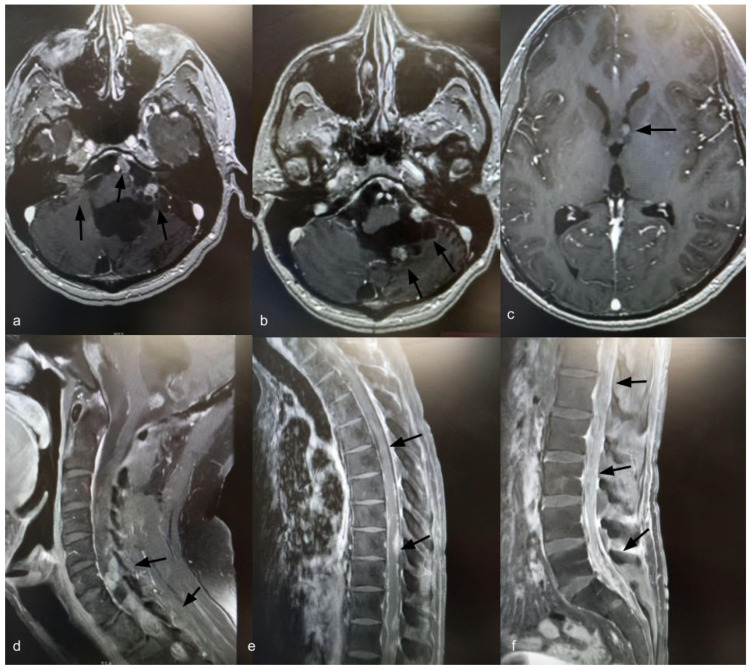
Axial T1-weighted brain MRI with gadolinium, 30 months after diagnosis of leptomeningeal dissemination, showing stable brain lesions (a–c) (indicated by black arrows). Sagittal T1-weighted spine MRI with gadolinium, 30 months after diagnosis of leptomeningeal dissemination, showing stable spinal enhancement and lesions (d–f) (indicated by black arrows).
